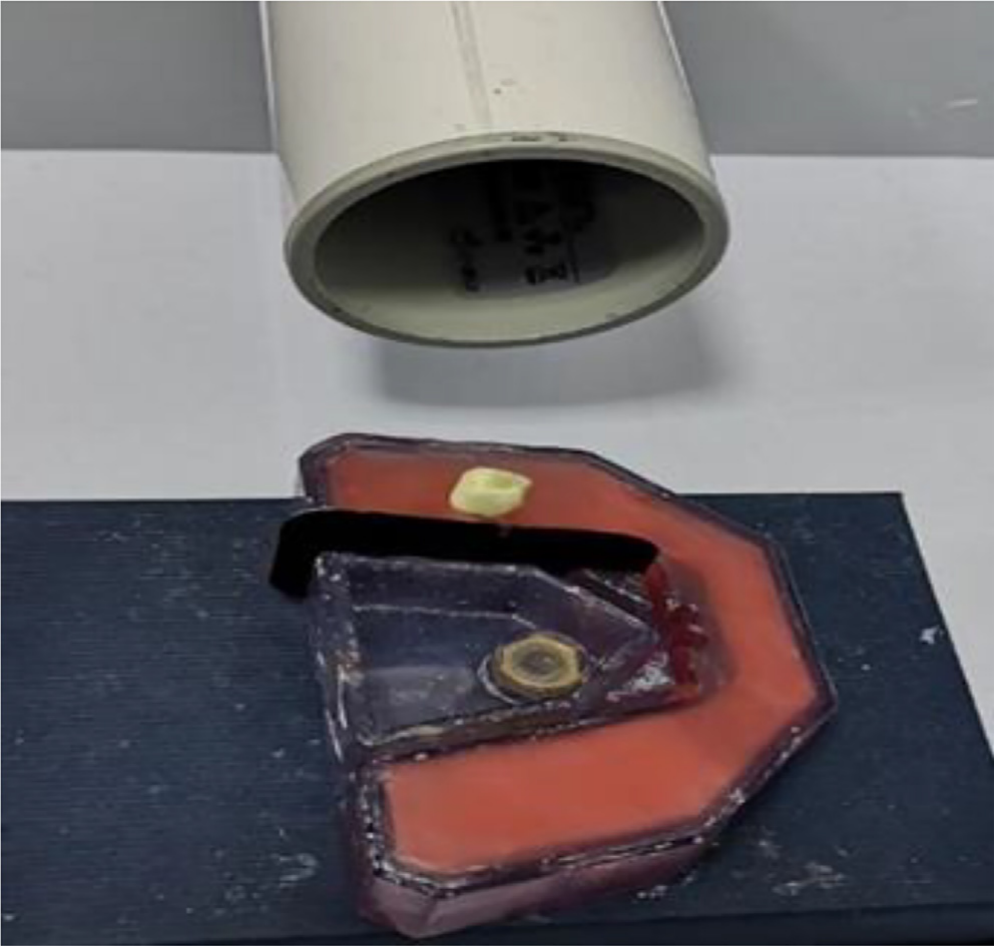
Radiographic technique with a mounted extracted tooth in wax cast.
